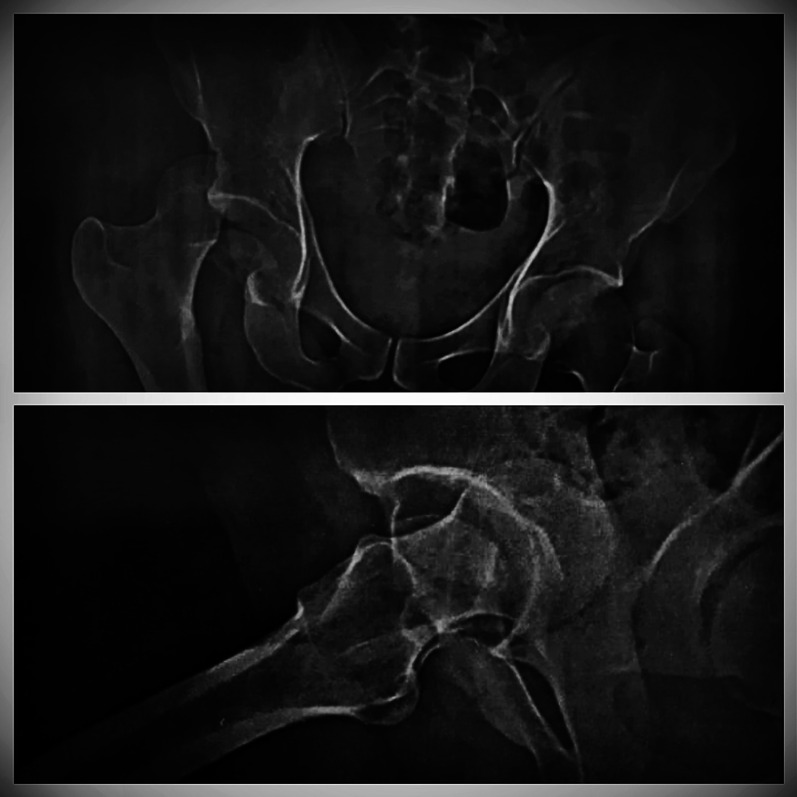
A, B Preoperative radiograph of a male 28-year-old patient with right hip dislocation and fracture of the femoral head (Pipkin type 2 fracture)
